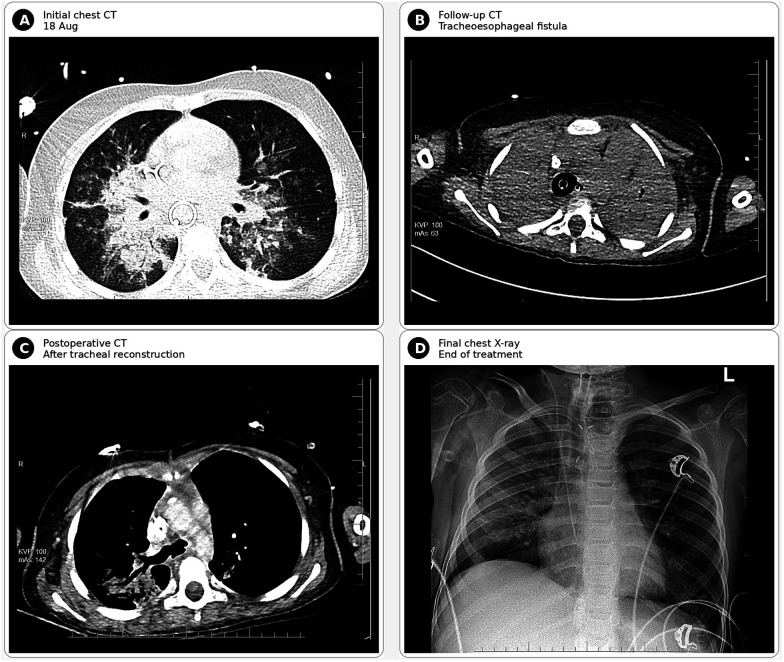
Serial imaging documenting the clinical course. (A) Contrast-enhanced chest computed tomography on hospital day 1 (August 18, 2023). Bilateral consolidation with predominantly perihilar distribution consistent with aspiration pneumonitis. A large, poorly defined soft-tissue density is visible in the posterior mediastinum, encasing the pulmonary arteries and compressing the left main bronchus. A covered esophageal stent is *in situ*; no definite tracheo-esophageal fistula is identified on this study. (B) Follow-up chest computed tomography demonstrating the tracheo-esophageal fistula with direct communication between the airway and the esophageal lumen at the carinal level, following resolution of the previously described mediastinal mass. (C) Postoperative chest computed tomography after tracheobronchial reconstruction with the esophageal muscular wall flap. The tracheal bifurcation is visible with patent origins of both main bronchi. Residual parenchymal changes and postoperative mediastinal alterations are present. (D) Posteroanterior chest radiograph obtained at the end of the intensive care stay (December 18, 2023), showing satisfactory bilateral lung expansion, tracheostomy cannula *in situ*, and percutaneous gastrostomy tube. No pneumothorax or significant pleural effusion.
